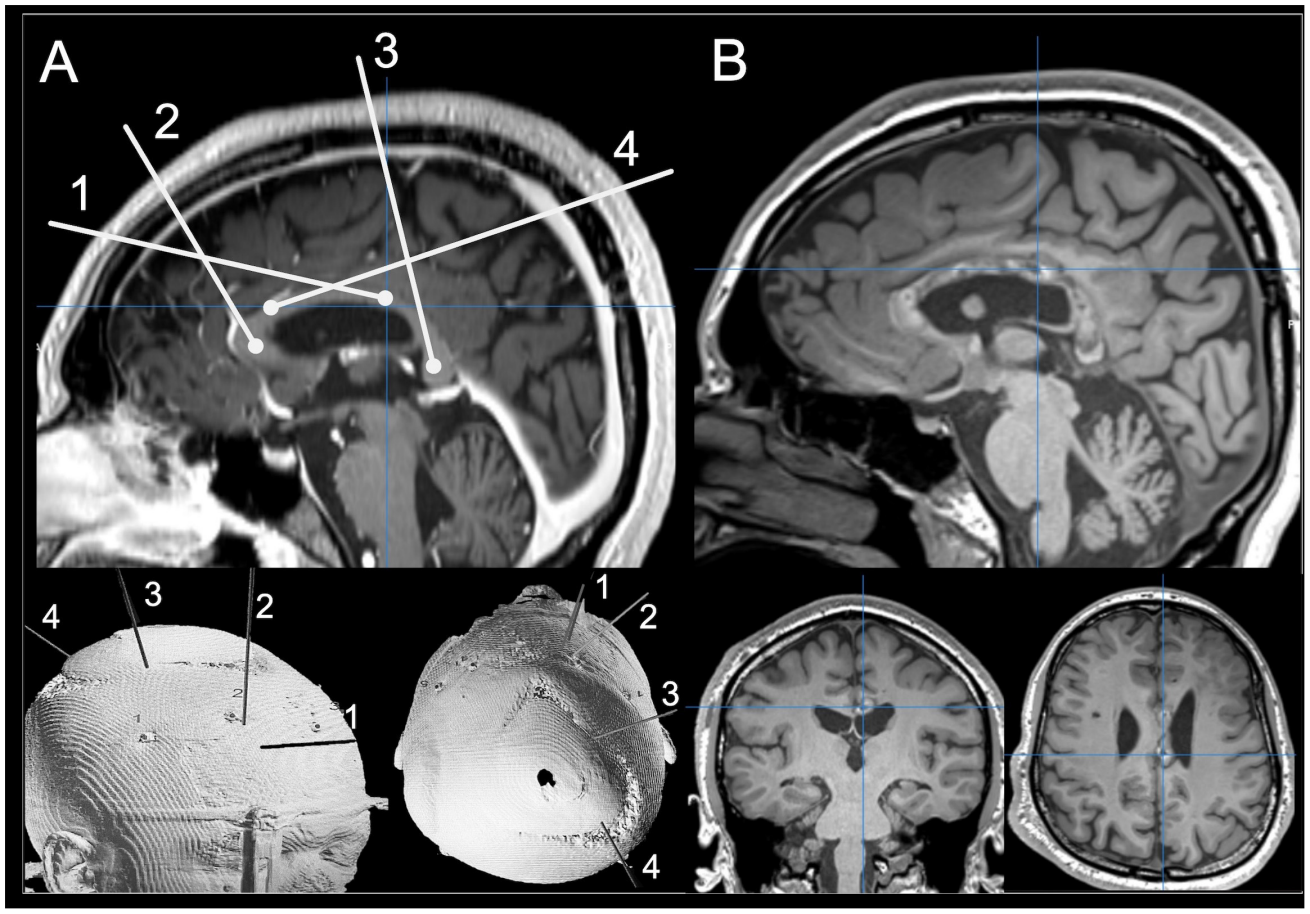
(A) Preoperative planning photo for a complete CCA using mid-sagittal T1-weighted MRI images and 3D rendering for the patient's skull. (B) Postoperative T1-weighted MRI images scans showing ablated tissue on mid-sagittal T1-weighted MRI images with coronal and axial views.
